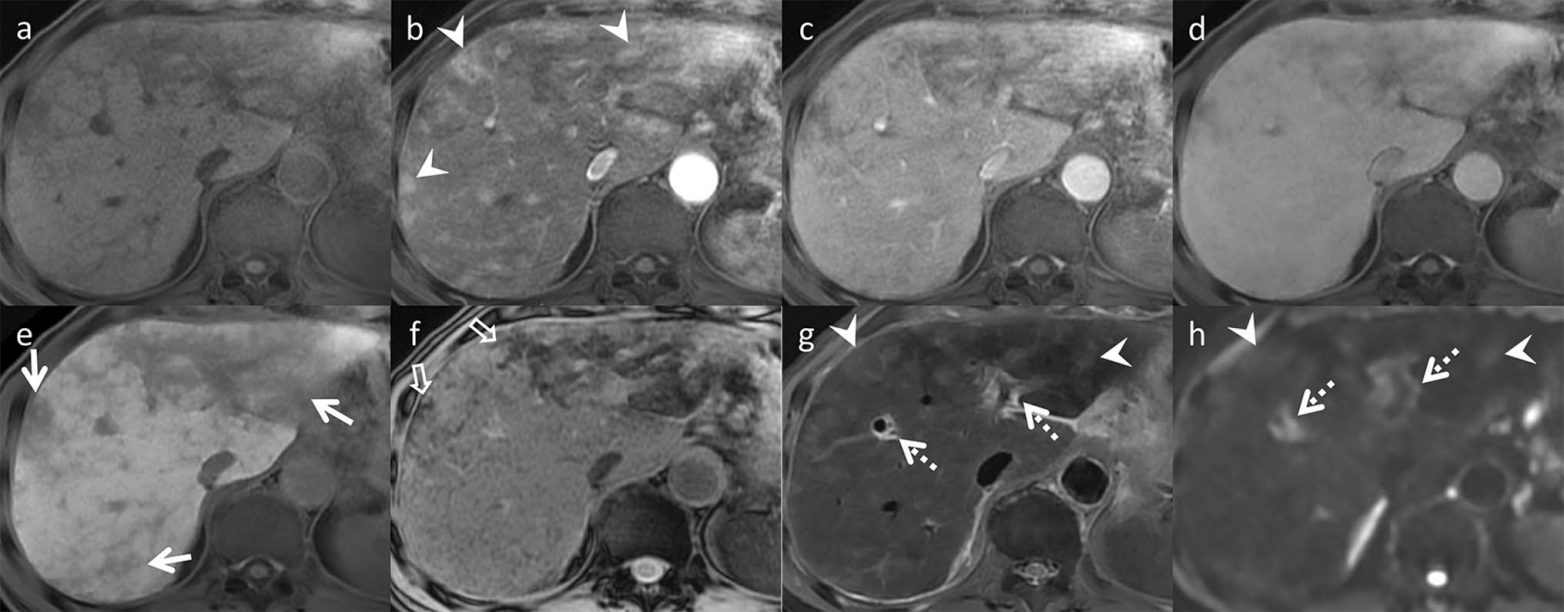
Sinusoidal obstruction syndrome (SOS) in an asymptomatic 65-year-old man, who was undergoing chemotherapy (S-1 and oxaliplatin) for esophagogastric junction cancer (same patient in Fig. 2). Gadoxetic acid-enhanced magnetic resonance imaging (MRI) (a pre-contrast, b arterial phase, c portal venous phase, d transitional phase, and e hepato-biliary phase) demonstrates diffuse hypo-intense reticulations in the liver, most obvious in the hepatobiliary phase (arrows). f. These areas show hypo-intensity on T2*-weighted imaging, which indicates hemorrhage (open arrows). Patchy heterogeneous enhancement in the arterial phase, heterogeneous hyper-intensity on g. fat-saturated T2-weighted imaging (fat-sat. T2WI), and h. diffusion-weighted imaging (DWI, arrowheads) with b value 800 s/mm^2^ are also evident. Periportal hyper-intensity is also observed in fat-sat. T2WI and DWI (dotted arrows). MRI is superior to computed tomography in the detection of SOS
